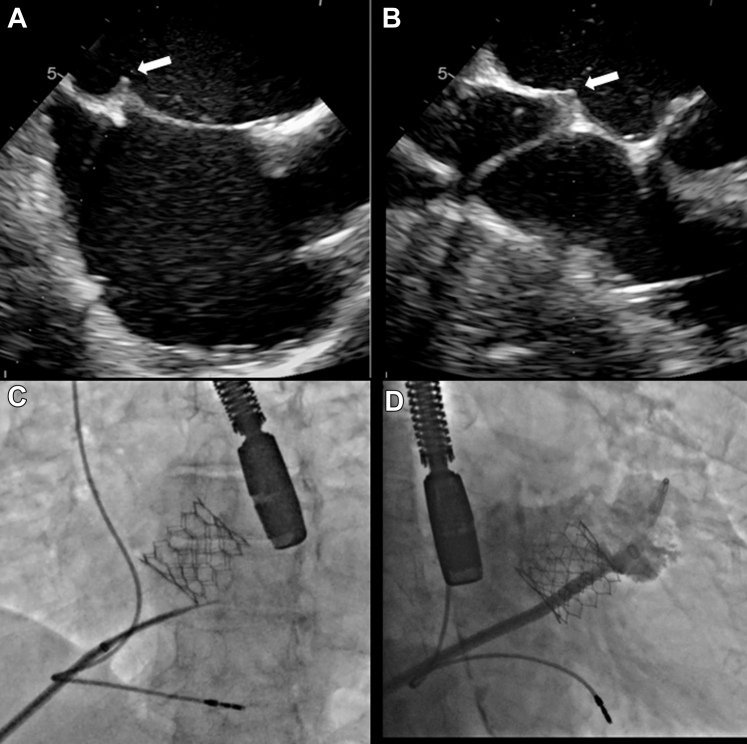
TEE-Guided Transseptal Puncture (A) TEE bicaval and (B) SAX views showing sheath tenting (white arrows). (C) X-ray fluoroscopy image with the septal puncture needle positioned. (D) LAA angiography. LAA = left atrial appendage; SAX = short axis; TEE = transesophageal echocardiography.
